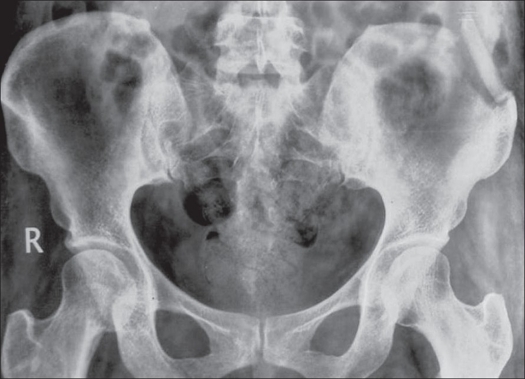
X-ray pelvis showing displaced rib graft
